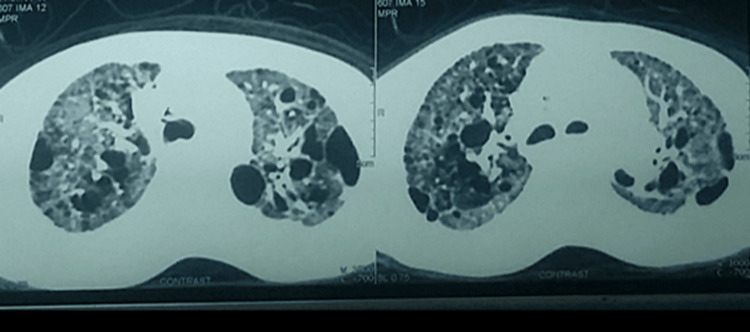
CT chest transverse section shows bilateral diffuse variable-sized lung cysts
